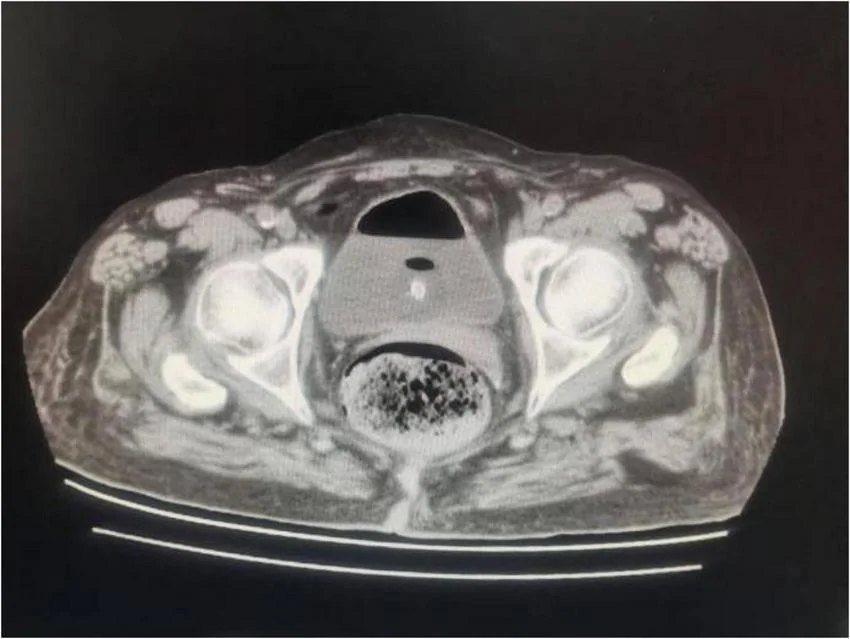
Gas shadows within the bladder visualized on the abdominal CT scan on July 3, 2025.
